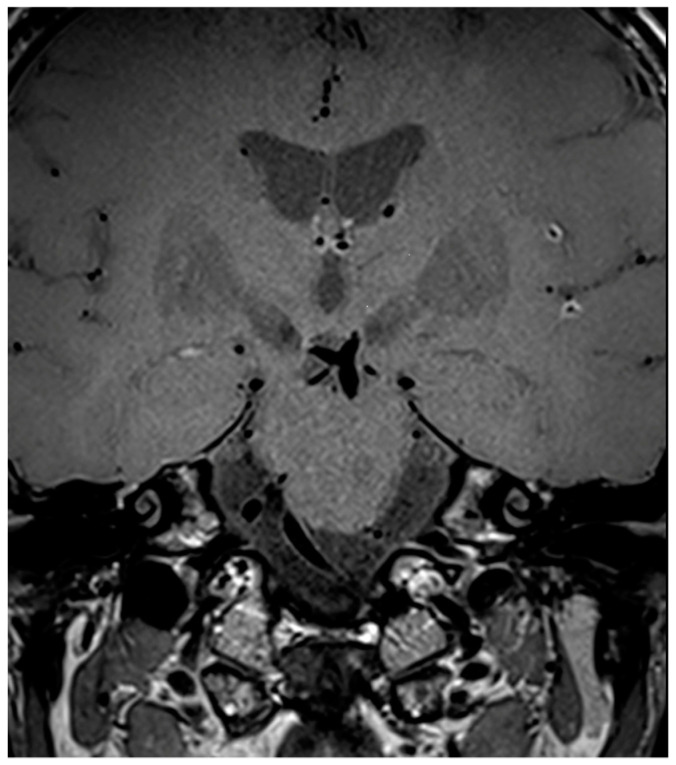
Three-dimensional SPACE T1-weighted VW image of a patient (M/49) with a diagnosis of infectious (luetic) CNSV. The image shows multiple (≥2) sites of concentric vessel wall enhancement of the distal branches of the right and left MCA.
